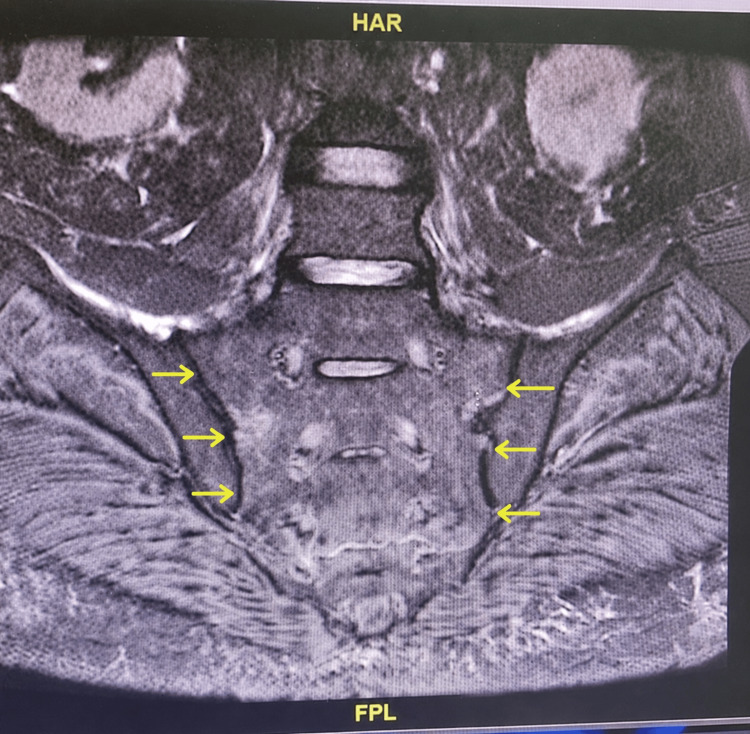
MRI of the sacroiliac joints The image demonstrates chronic structural changes, including erosions and sclerosis at the sacroiliac joints (arrows), without bone marrow edema or active inflammatory features, consistent with inactive (burned-out) axial spondyloarthritis MRI: magnetic resonance imaging
